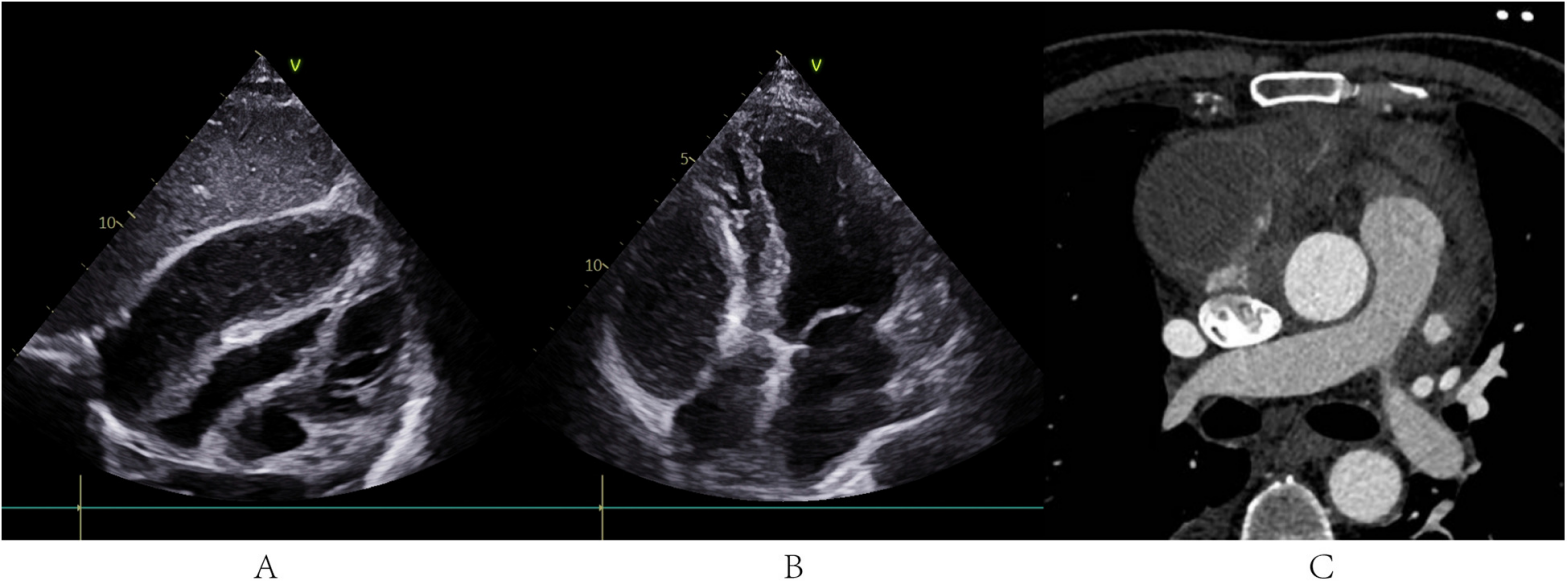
TTE and cardiac CT before PTCA guidewire-based pericardiocentesis. (A,B) TTE revealed a loculated gelatinous effusion in the posterior wall of the right pericardial cavity compressing the right atrium and ventricle, with pericardial adhesions. CT confirmed pericardial thickening, blurred pericardiophrenic fat planes, and a septated purulent collection adjacent to the right atrium.
